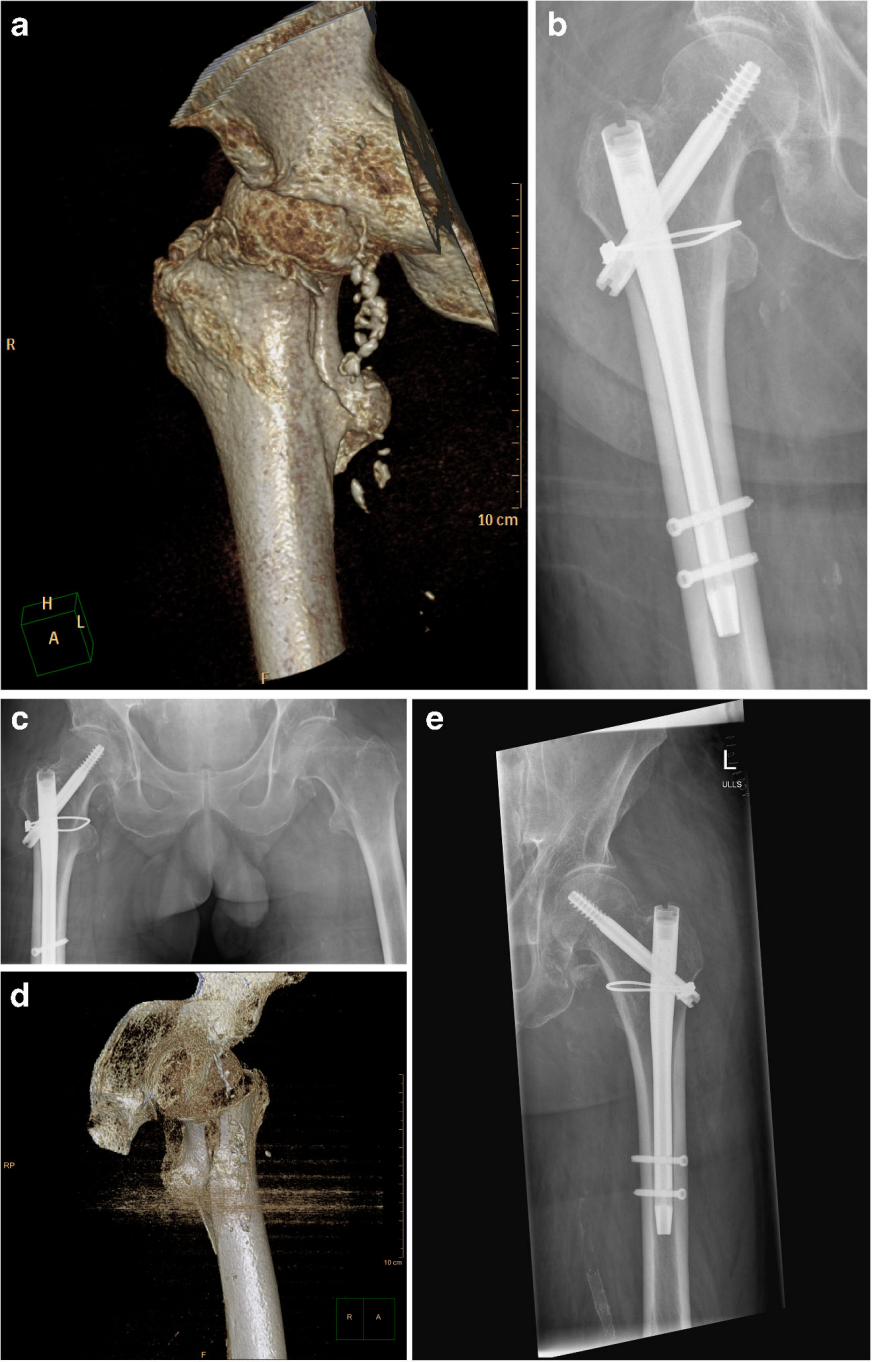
a AO/OTA A1.3 trochanteric fracture on the right side in a 72-year-old patient after fall from a standing position. b Open reduction and internal fixation (ORIF) was carried out on the traction table using a cephalomedullary nail and one auxiliary cerclage. c Three years later, a fall from a standing position in the same patient resulted in an identical AO/OTA A1.3 trochanteric fracture on the left side. Simultaneously, the X-ray provided a 3-year follow-up demonstrating complete fracture healing on the right side. d In terms of the complex fracture configuration, an additional CT scan was performed which demonstrated a sagittal fracture line resulting in the decision to use an additional cerclage. e Post-operative X-ray demonstrated the situation following ORIF on the traction table with cephalomedullary nail and one additional cerclage
